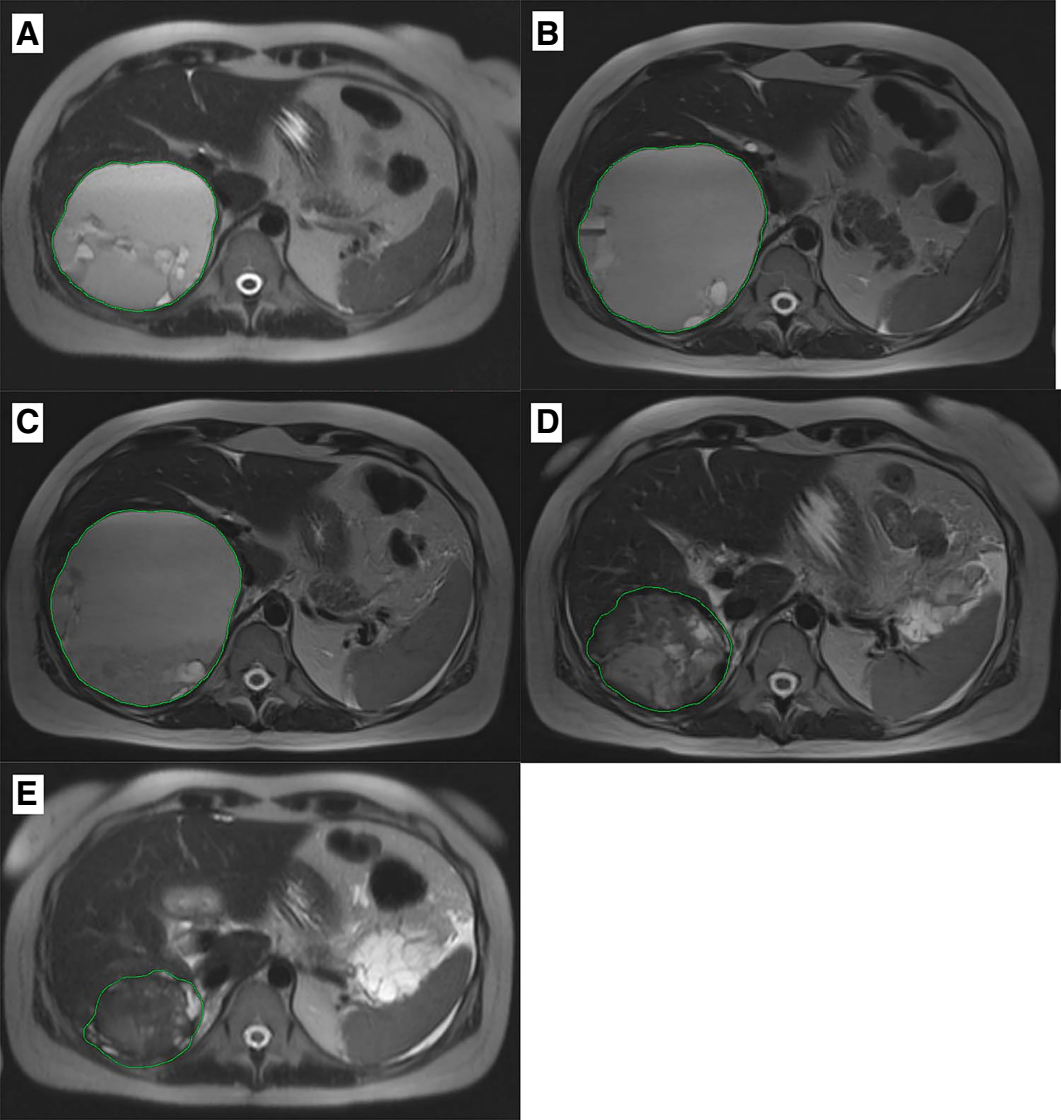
Cystic-solid neoplasm on the lower contour of the liver (neoplasm #1). (*A*) MRI from 03.2016; (*B*) MRI from 06.2016; (*C*) MRI from 09.2016; (*D*) MRI from 10.2017; (*E*) MRI from 03.2018.
